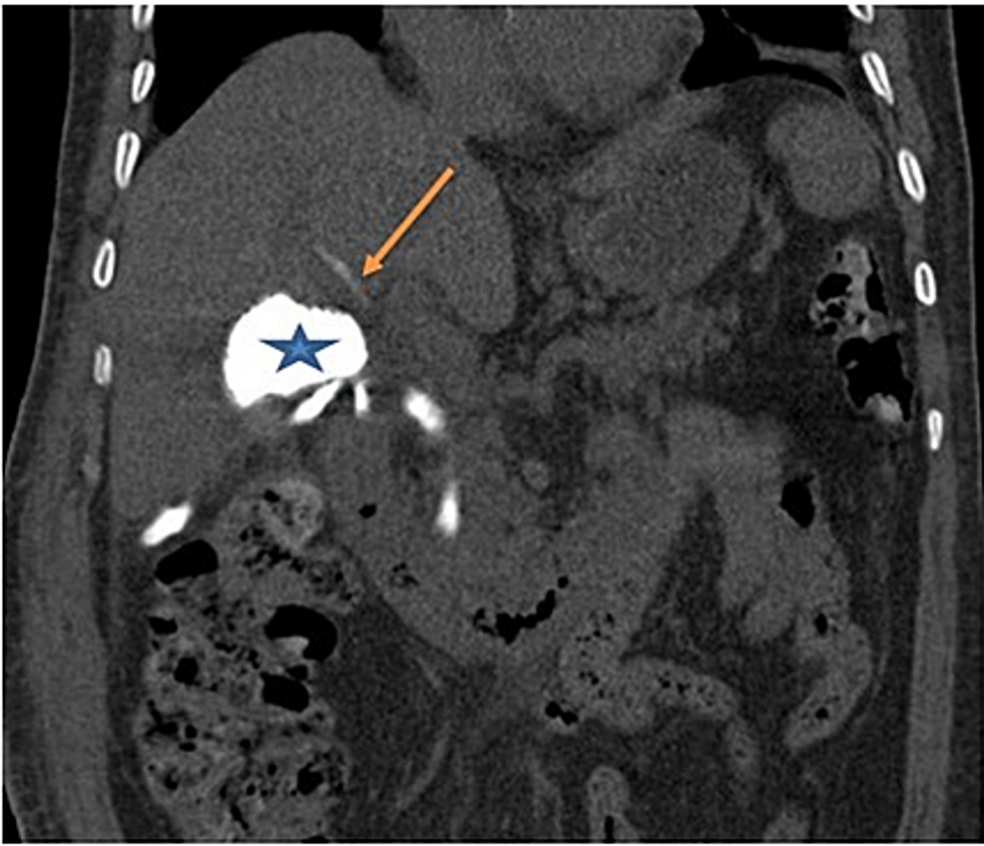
Non-contrast-enhanced CT scan after endoscopic retrograde cholangiopancreatography The image shows biliary leakage at the hilar level (blue star) with opacification of an intrahepatic bile duct at segment IV: duct of Luschka (orange arrow) CT: computed tomography
